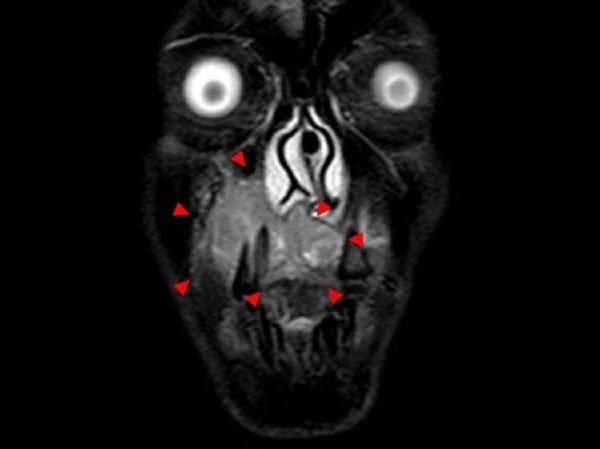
Preoperative magnetic resonance imaging shows the lesion in the right maxilla (arrows).
